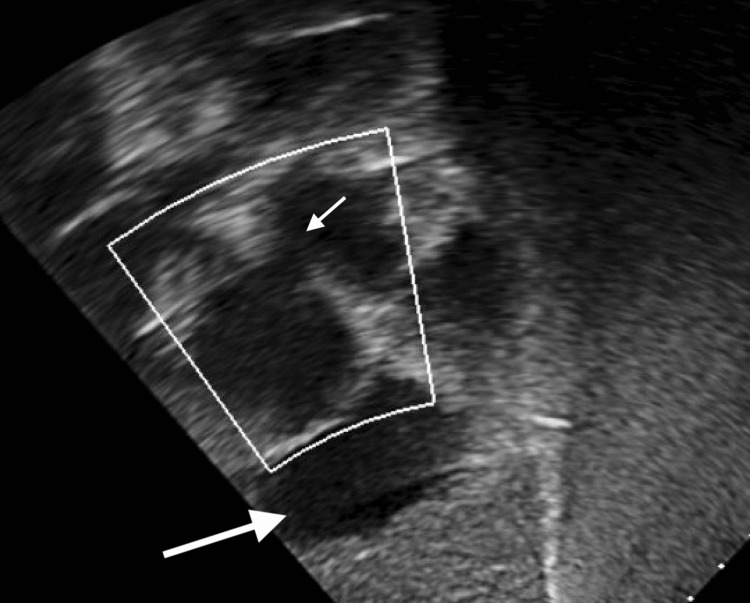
Transthoracic echocardiogram demonstrating a large secundum atrial septal defect between the left and right atria (small arrow) and right ventricular enlargement due to shunting of blood flow to the right side of the heart (large arrow).
